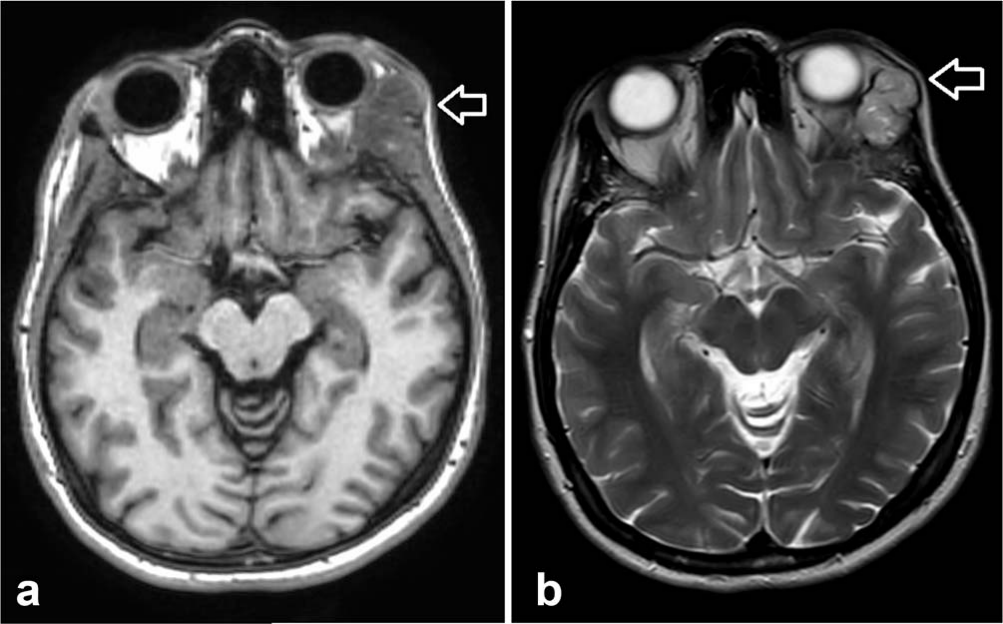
Orbit MRI showed a lobulated, well-defined lesion, hyperintense to intermediate on T2W (b) and hypointense on T1W (a) structures with cystic changes, measuring 2.3 × 1.9 cm and 2.6 × 1.6 cm, compared with the previous study, the lesions have increased in size.
